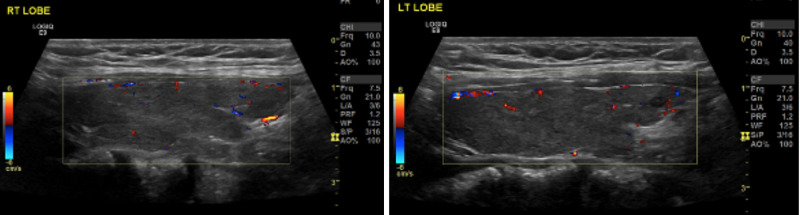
Duplex Doppler ultrasound of left and right lobes, showing a bilateral decrease in vascularity.
